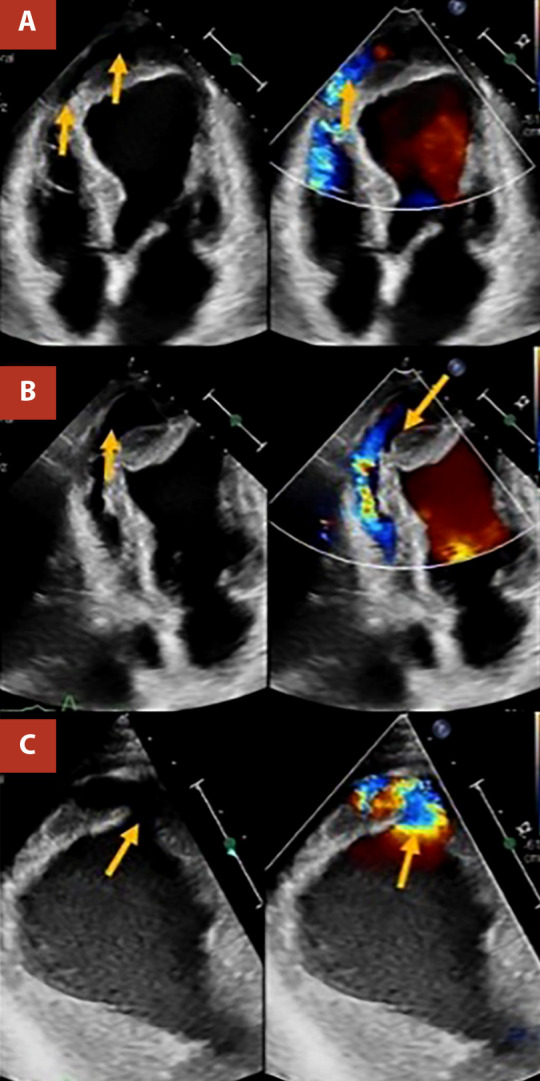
Transthoracic echocardiography on admission. Dilated left chambers with moderate concentric hypertrophy. Left ventricular ejection fraction of 34%. The apical myocardium is divided into two layers (intramyocardial dissection), demonstrated by an anechoic space with internal flow (A), which communicates with the right ventricle through apical septal rupture (B). Apical ventricular septal rupture measuring 3 mm (C).
